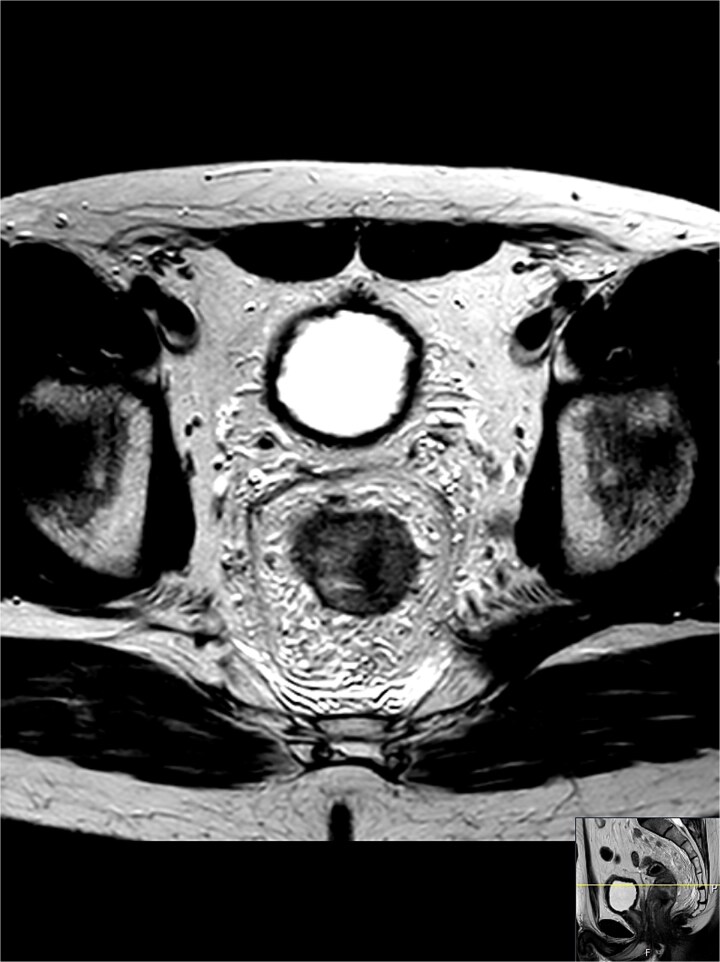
A cross-section of the MRI shows a rectal tumour mass located between 4 and 8 o’clock; the shortest distance to the mesorectal fascia was 4 mm from the tumour and 1 mm from a lymph node that appears malignant; there were multiple enlarged lymph nodes in the area, also outside the mesorectum with possible venous infiltration; radiologically categorized as T3bN2.
